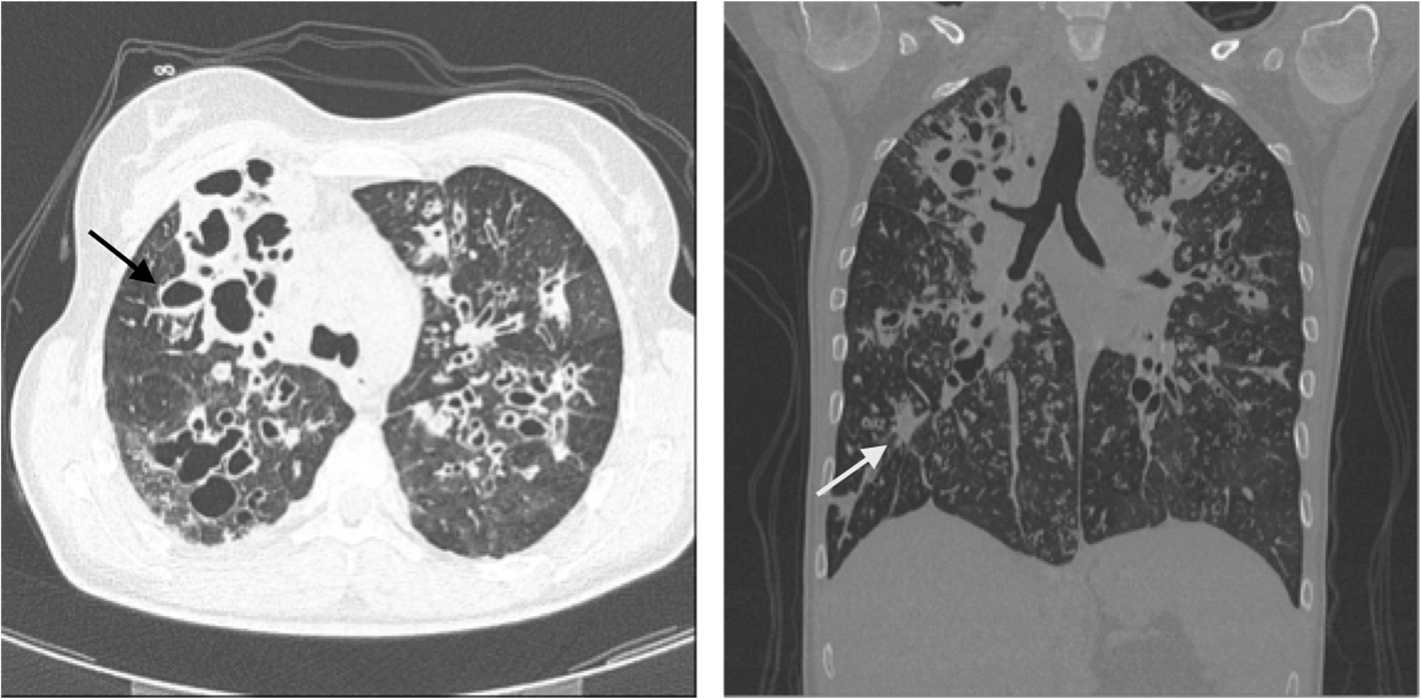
High Resolution Computed Tomography (HRCT) of the Patient 1′ Lungs. A) Axial and B) Coronal HRCT images showing severe bilateral bronchiectasis, mainly in the upper lobes (black arrow), with associated bronchial wall thickening and mucus retention in bronchial lumen. Bilateral ground-glass opacity with areas of consolidation (white arrow)
